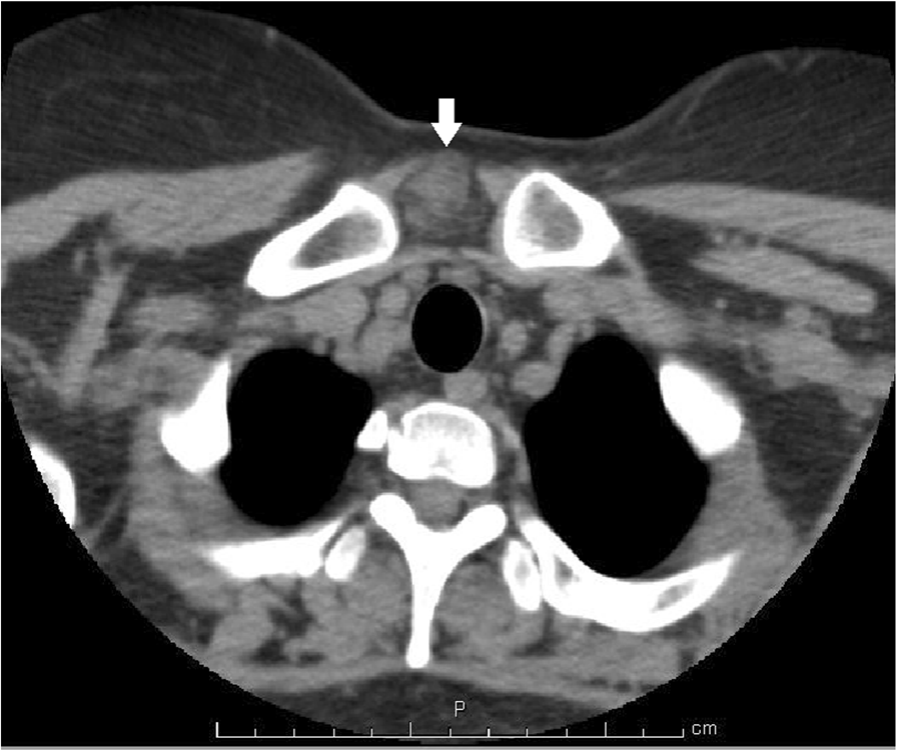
The neck non-enhanced computed tomography scan revealed that the mass in the suprasternal subcutaneous tissue had the mixture components of soft tissue and fat density (arrow)
